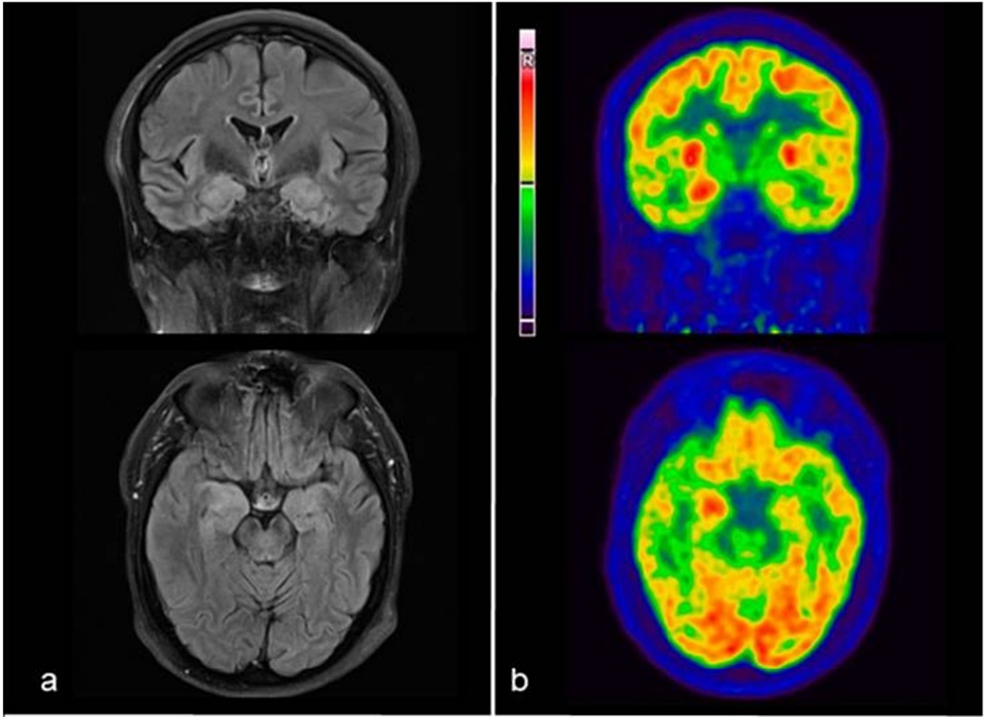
Example of anti-CASPR2 encephalitis demonstrated (a) MRI-3T, T2-FLAIR axial and coronal slices showing medial temporal hyperintensity as well as swelling, predominantly on the right side; (b) Corresponding 18F-FDG-PET/CT axial and coronal slices showing right medial temporal hyperactivity (licensed under CC BY-ND 4.0) [72] CASPR2 = contactin-associated protein-like 2, FLAIR = fluid-attenuated inversion recovery, 18F-FDG-PET = 18F-fluorodeoxyglucose-positron emission tomography
